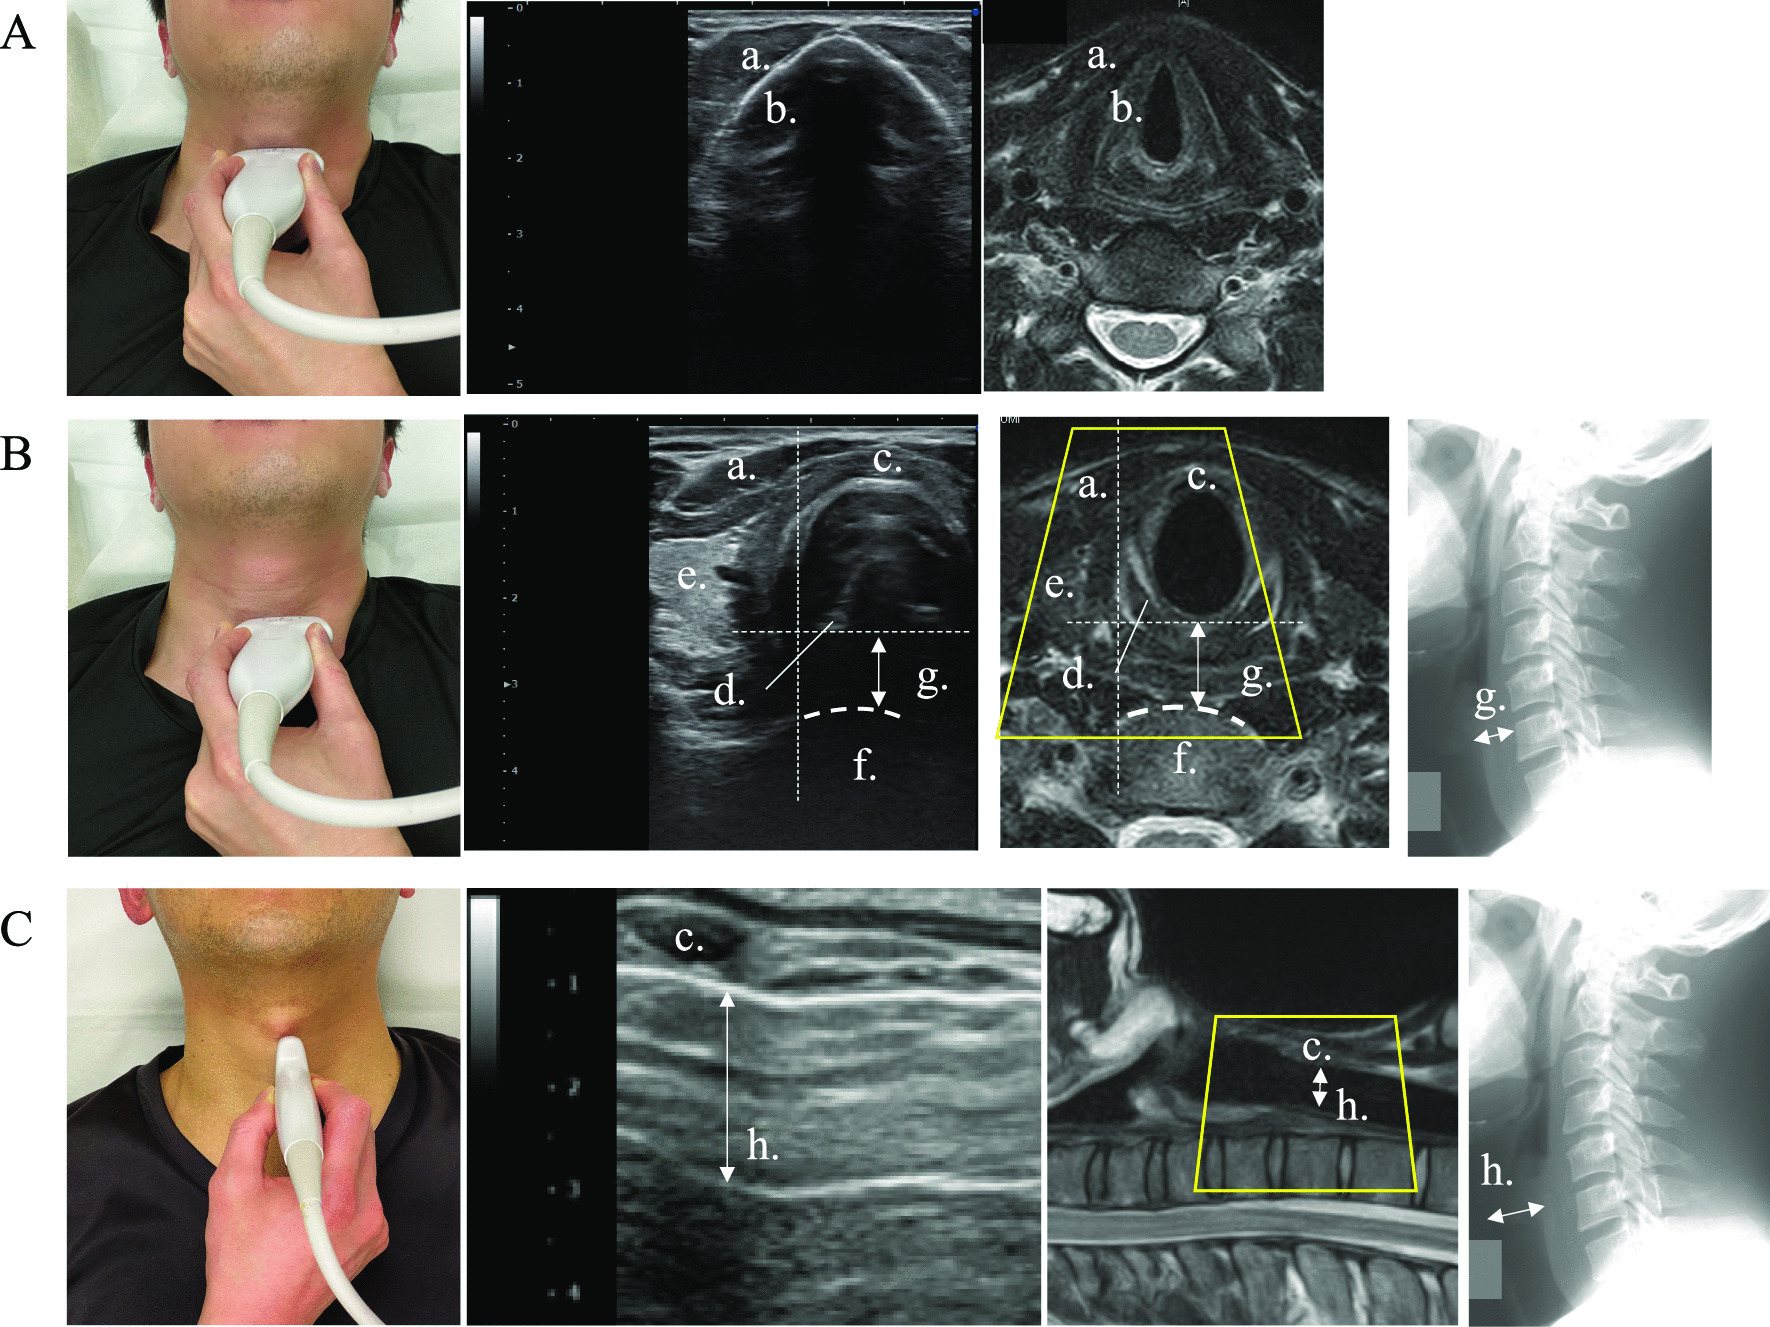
Macro photograph, ultrasound image, and magnetic resonance image A, B: Thyroid cartilage and cricoid cartilage in the transverse plane. C: Cricoid cartilage and tracheal cartilage in the sagittal plane a: sternohyoid muscle, b: thyroid cartilage, c: cricoid cartilage, d; arytenoid cartilage, e: thyroid gland, f: C6 vertebral body, g: the thickness of the prevertebral soft tissue, h: upper airway
